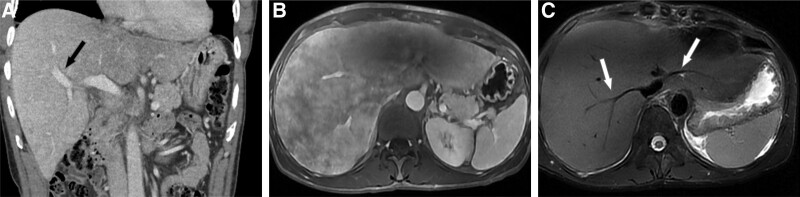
(A) Coronal computed tomography obtained during the portal venous phase shows periportal edema (black arrow) and mildly heterogeneous, mottled parenchymal enhancement in the liver. The volume of the left hepatic lobe is decreased and the volume of the right hepatic lobe is increased. (B) After 3 months, contrast-enhanced T1-weighted axial magnetic resonance (MR) obtained during the portal venous phase reveals more extensively heterogeneous, mottled parenchymal enhancement in the liver. The hypotrophy of the left liver and hypertrophy of the right liver is still noted. In addition, the surface contour of the liver is slightly lobulated. (C) Respiratory-triggered fat-suppressed T2-weighted image shows decreased hepatic vein diameter (white arrows). These MR findings suggest chronic liver disease.
